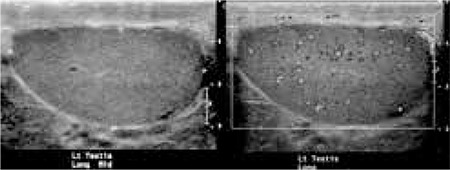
Ultrasound shows mildly heterogeneous echotexture in the left testis with mild hypervascularity and thickened scrotal skin
